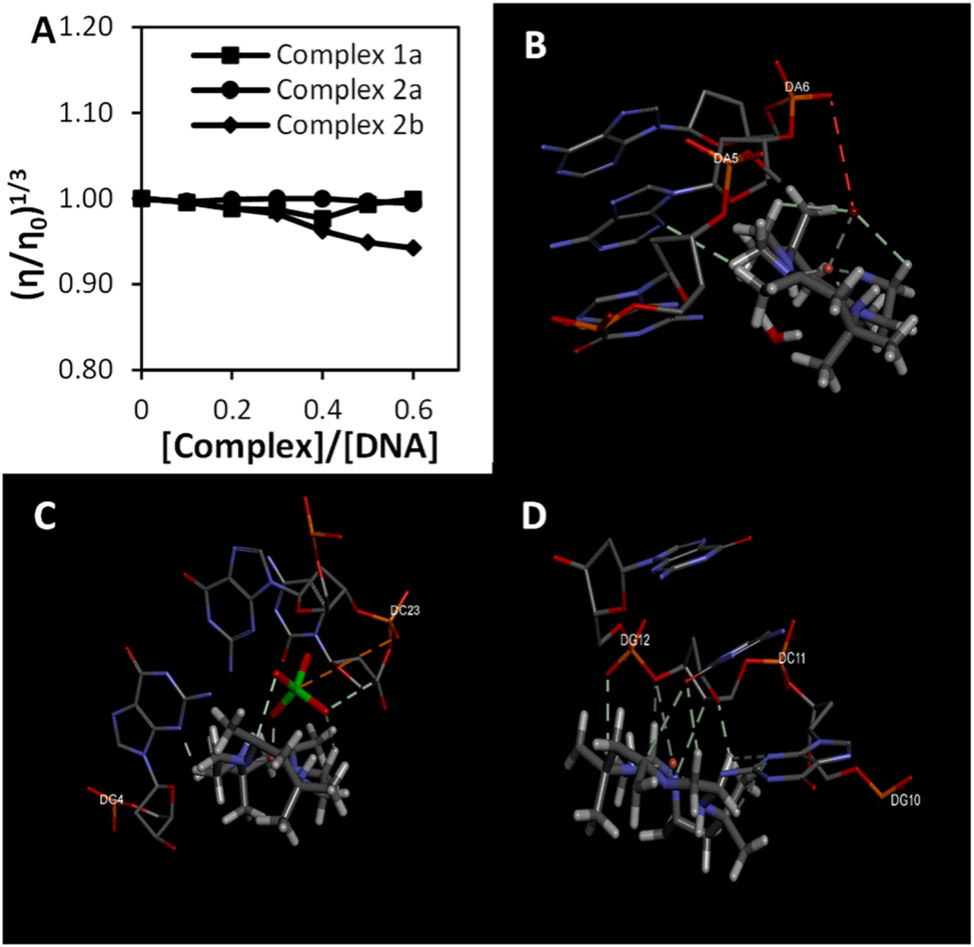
The binding study evaluations using viscosity and computational study (a) effects of the increasing amount of 1a, 2a, and 2b complexes on the relative viscosity of CT-DNA (b–d) molecular docked structures of the complex with DNA dodecamer duplex of sequence d(CGCGAATTCGCG)_2_ (PDB 1D: 1BNA). (b) Complex 1a, (c) complex 2a, (d) complex 2b.
